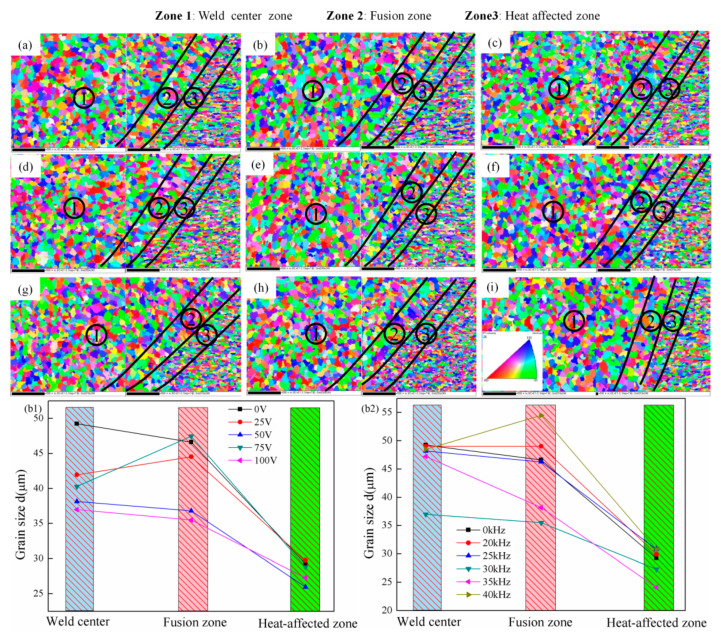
Grain orientation at different ultrasonic excitation parameters from (a) 0 V, 0 kHz, (b) 25 V, 30 kHz, (c) 50 V, 30 kHz, (d) 75 V, 30 kHz, (e) 100 V, 30 kHz, (f) 100 V, 20 kHz, (g) 100 V, 25 kHz, (h) 100 V, 35 kHz, (i) 100 V, 40 kHz; and Grain size from different: (b1) ultrasonic excitation voltages, and (b2) ultrasonic excitation frequency.
